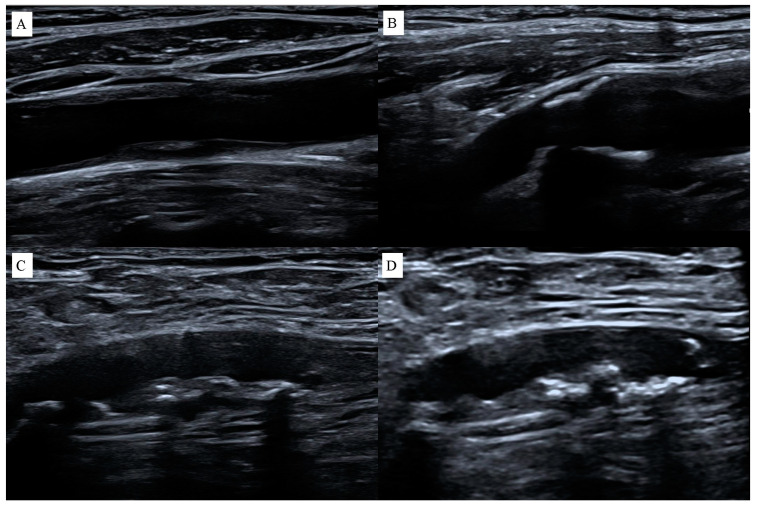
Atherosclerotic plaques on ultrasound examination: (A) heterogeneous plaque in the common carotid artery, (B) homogeneous hyperechoic plaques in the carotid bulb, (C) heterogeneous plaque in the superficial femoral artery, (D) hyperechoic plaque in the superficial femoral artery.
